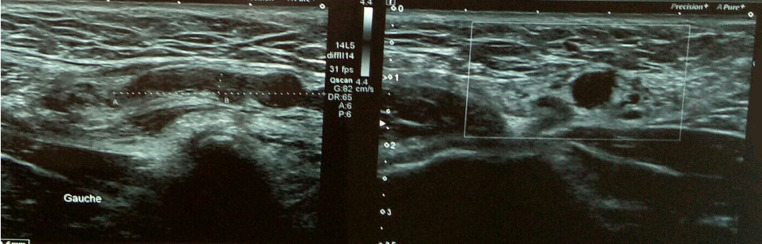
ultrasonography demonstrated tubular structure hypoechoic non compressed consistent with thrombus
